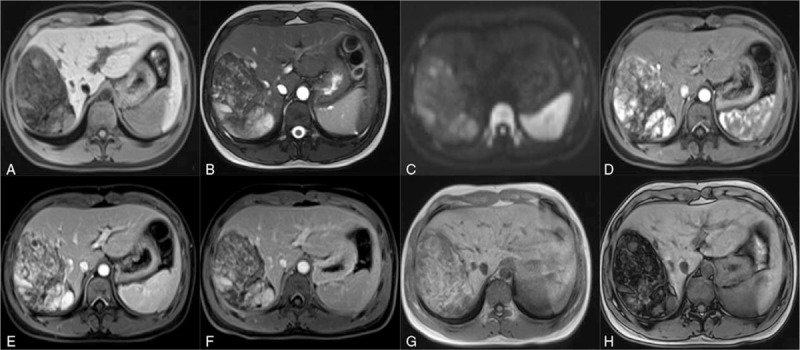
(A) Axial T1-weighted MRI shows a heterogeneous hypointense mass in the right lobe of the liver.(B) Axial T2-weighted MRI shows again the heterogeneous hyperintense mass with a clear boundary. (C) Diffusion-weighted imaging shows that the mass is hyperintense. (D) The arterial phase shows that the mass was initially obviously heterogeneously enhanced after the administration of gadolinium. (E) Both the portal phase and (F) delayed-phase images show that the enhancement degree of the lesion was decreased but still visible. (G)In-phase and (H) out-phase images show signal decline within the lesion. MRI = magnetic resonance imaging.
